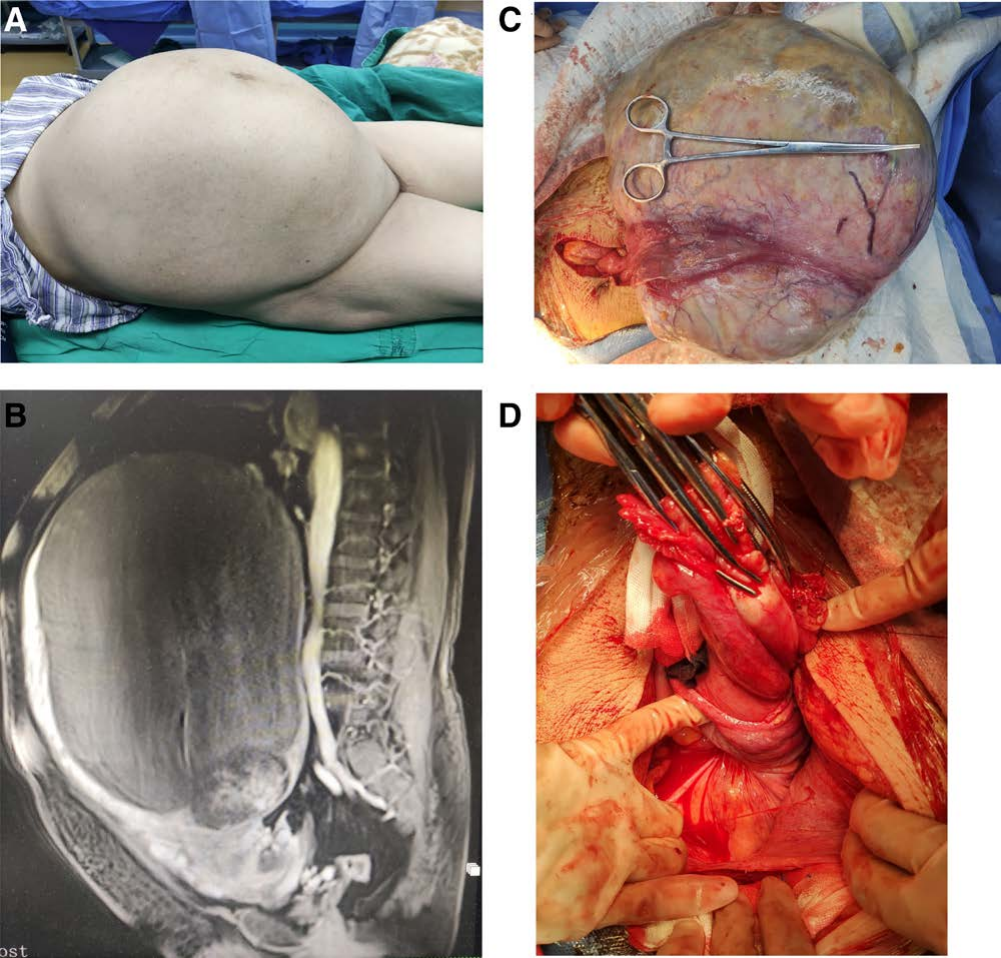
Photos taken from the patient showing the giant mass and torsion uterus. (A) Distended abdomen of the patient. (B) Enhanced MRI showed the giant mass and torsion uterus. (C) Giant mass out of the abdominal cavity. (D) Torsion uterus. MRI = magnetic resonance imaging.
